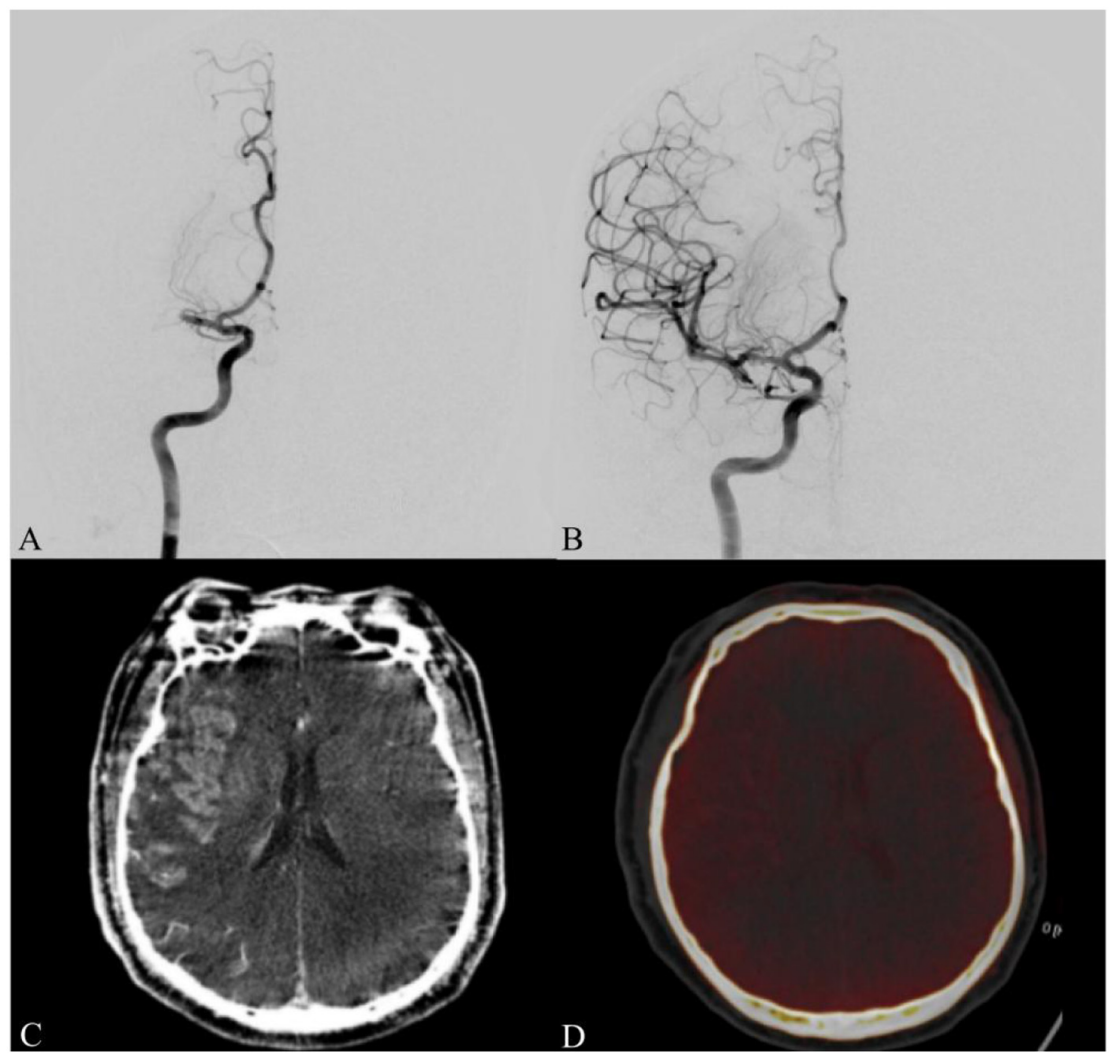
A patient with occlusion of the right middle cerebral artery M1 segment. (A) Preoperative digital subtraction angiography (DSA) shows no antegrade flow (mTICI 0). (B) Postoperative angiography demonstrates complete reperfusion (mTICI 3) following mechanical thrombectomy. (C) Immediate post-thrombectomy Dyna-CT images showing contrast agent extravasation and retention in the brain parenchyma. (D) Post-thrombectomy dual-energy CT confirmed that the ipsilateral hyperdensity represented contrast extravasation, with mild cerebral edema and slight leftward midline shift. DSA, digital subtraction angiography; mTICI, modified thrombolysis in cerebral infarction.
